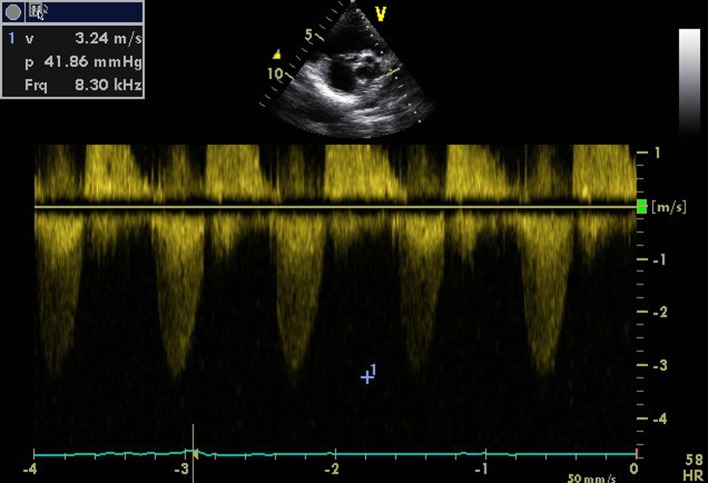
Transthoracic echocardiogram of parasternal short axis view showing pulmonic stenosis and regurgitation.
